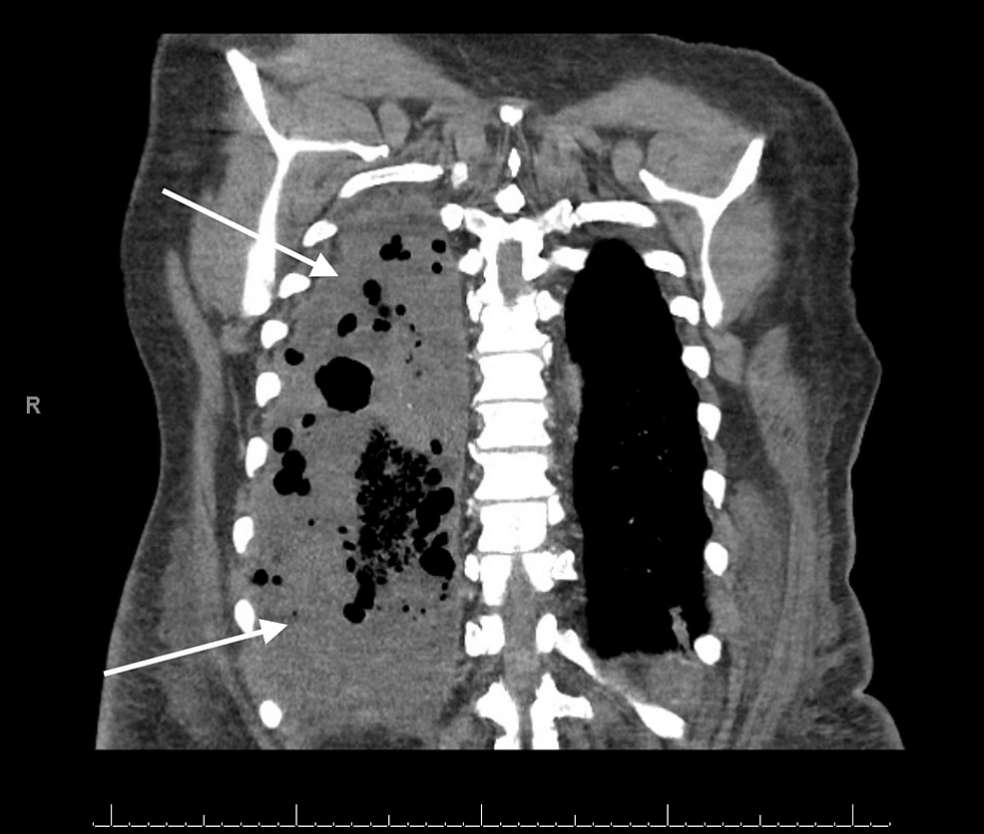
Coronal view of the chest CT showing multilobar opacities on the right side (arrows) depicting the extent of necrotizing pneumonia
